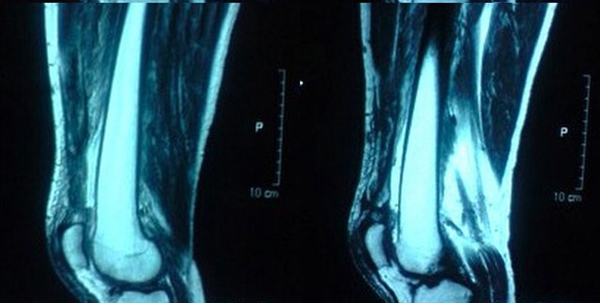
Magnetic resonance images of the knees. The magnetic resonance scan revealed disruption of the quadriceps at a level of 1.5 cm from the insertion into the patella on both sides, right more extensive than left. There was thickening of the quadriceps on both sides.
